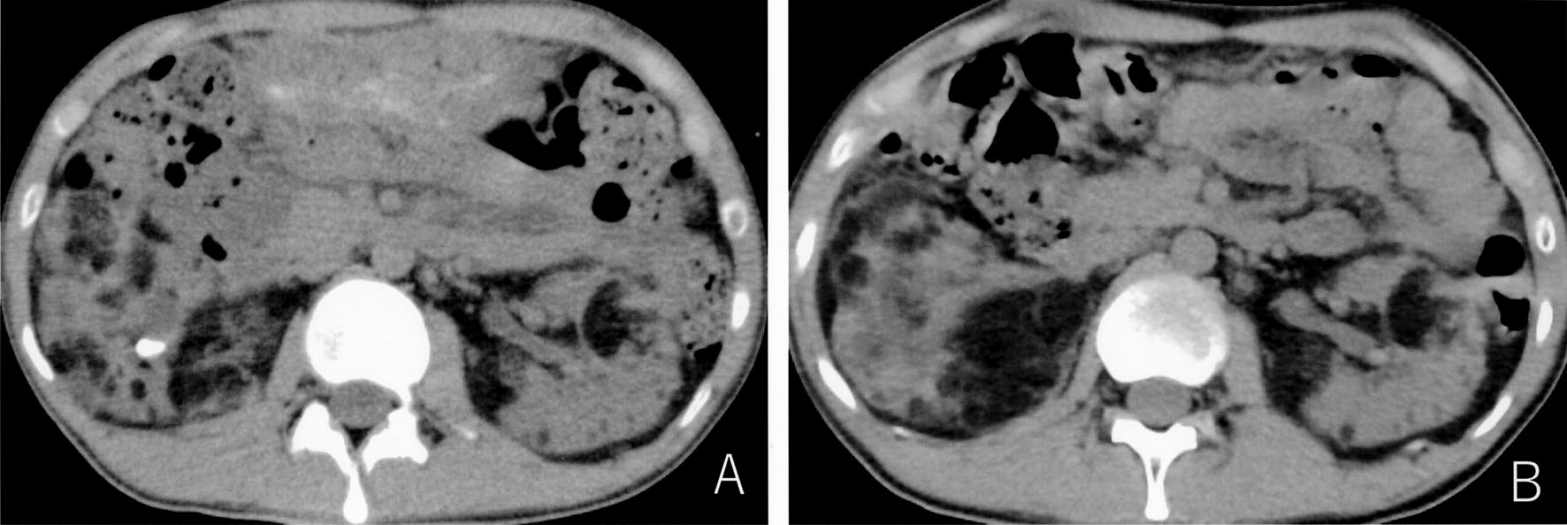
Axial CT of the kidney. a A 13 mm stone can be seen in the right kidney. Multiple AMLs are observed around the stone. b The stone was completely removed after transurethral lithotripsy
